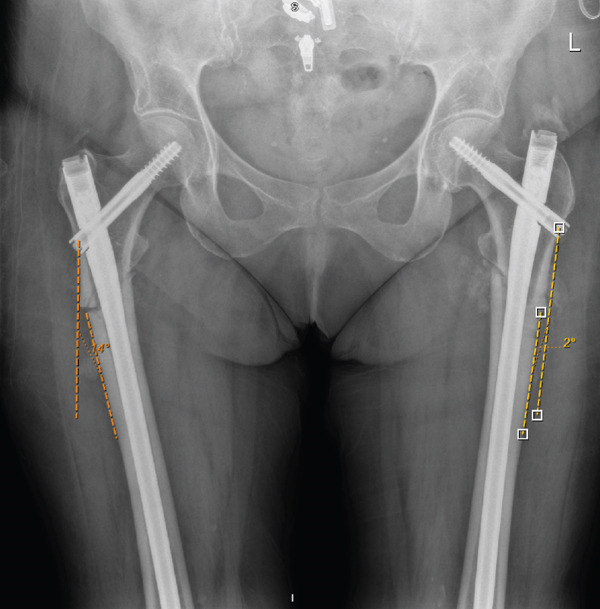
Early postoperative anteroposterior radiographic imaging showing cephalomedullary nailings for initial bilateral femoral fracture fixation with evidence of callus formation. Noted left femur fixation in valgus alignment (measured 2°) with a more medial entry point and right femur fixation in varus (measured 14°) with a lateral entry point.
